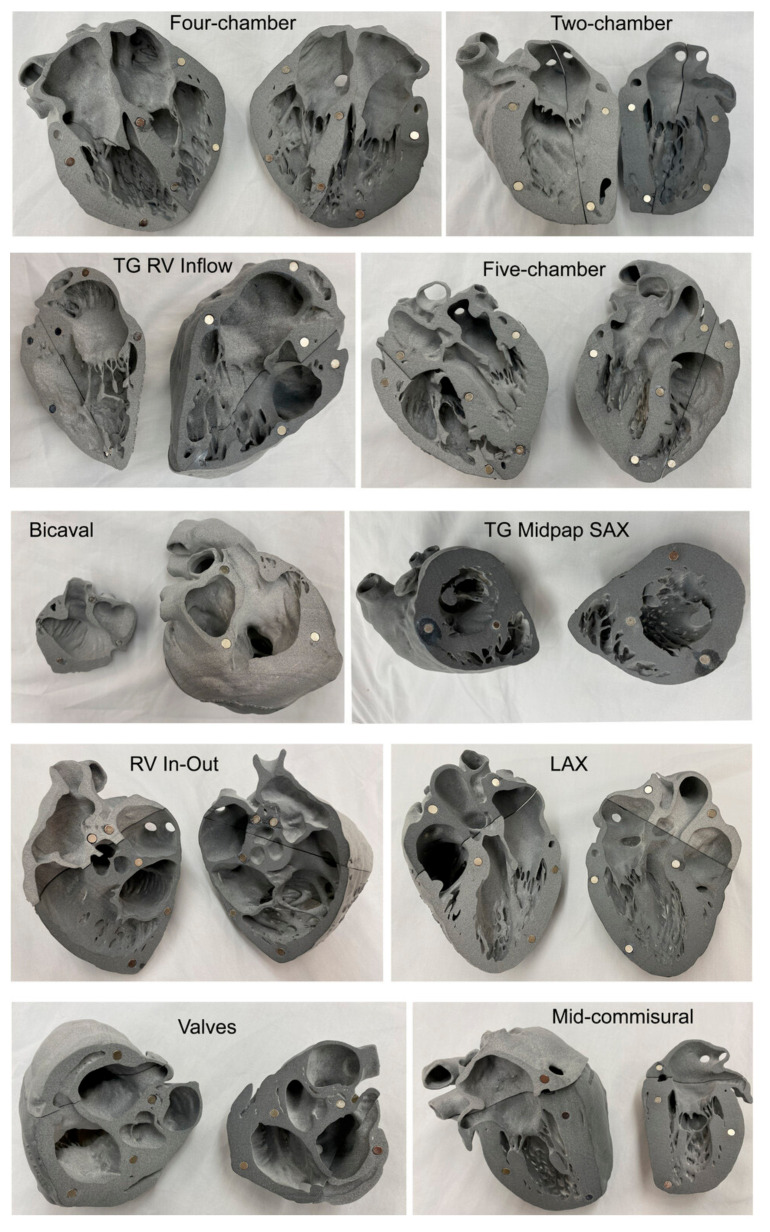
High-resolution fusion powder 3D-printed heart models representing the transesophageal echocardiography (TEE) American Society of Echocardiography (ASE)-recommended views. Each row represents two corresponding planes on each model that have been labeled accordingly. LAX, long axis; RV, right ventricle; SAX, short axis; TG, transgastric. Reprinted with permission from Arango et al. [103].
